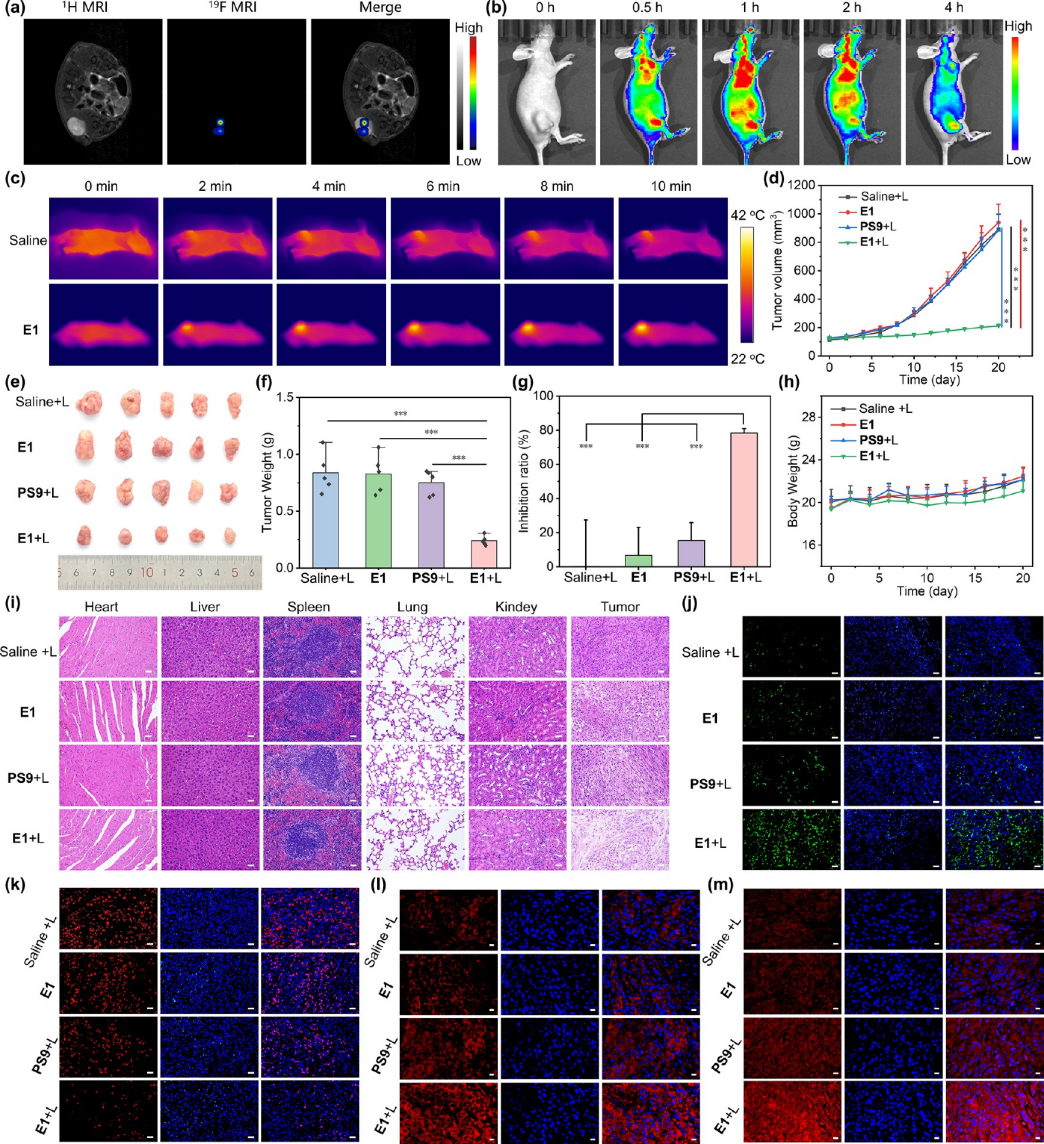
^1^H/^19^F MRI (a), whole-body FLI (b), and thermal
